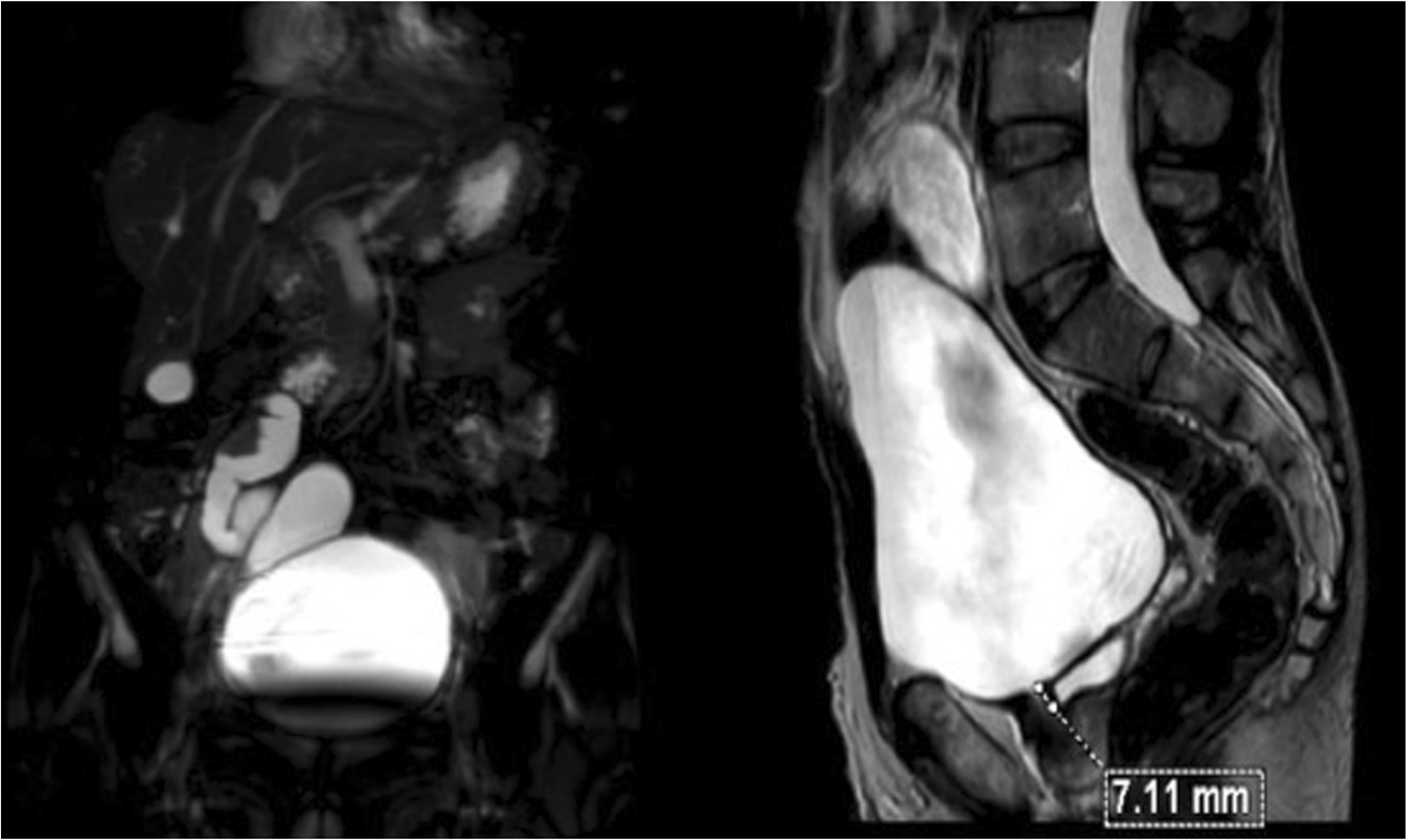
Uro-magnetic resonance imaging: tumorous ureter ends in the right prostatic lobe
